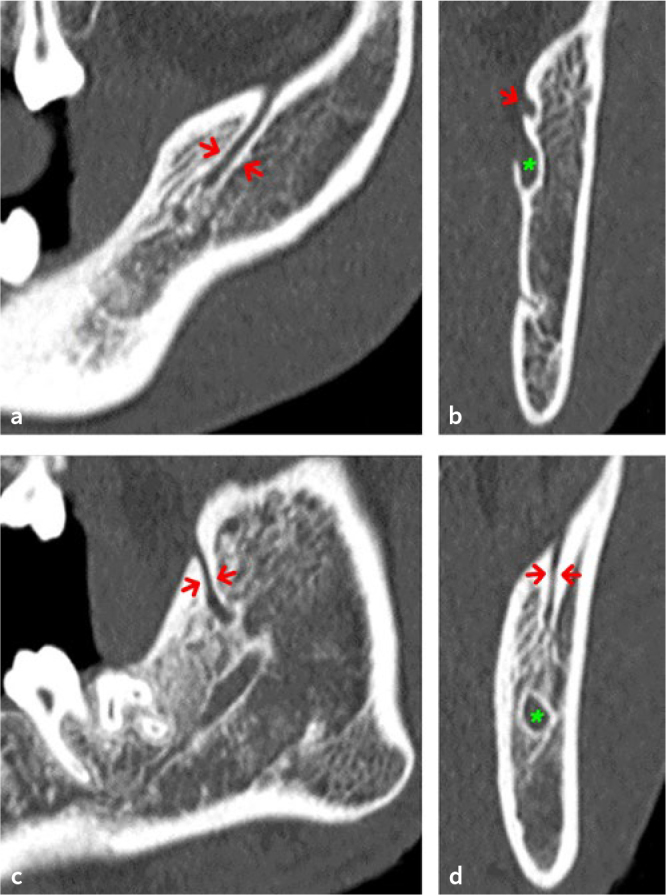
CBCT images of a temporal crest canal (TCC): a. Sagittal CBCT slice showing initial course of TCC (red arrows); b. coronal CBCT slice showing the mandibular foramen (green asterisk) and the accessory foramen (red arrow); c. sagittal CBCT slice showing the last part of TCC (red arrows); d. coronal CBCT slice showing the TCC opening at a bony foramen (red arrows) and the mandibular canal (green asterisk).
